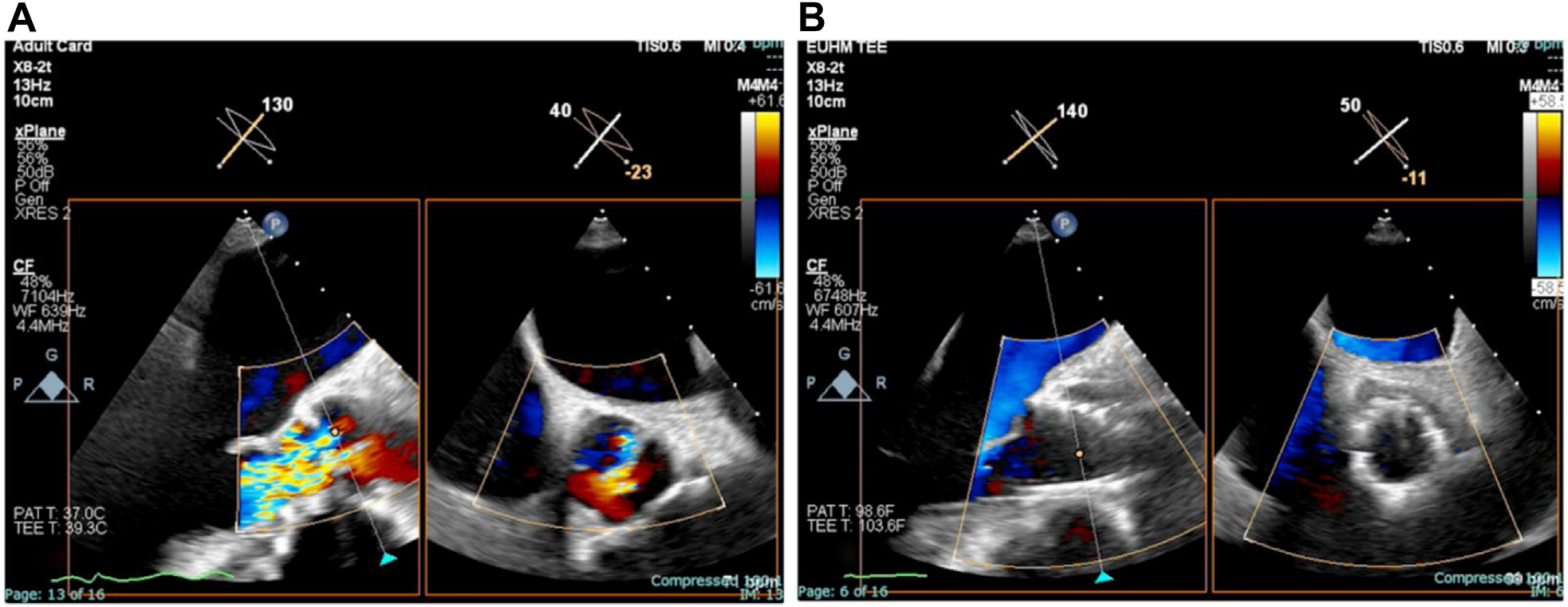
Transesophageal echocardiography images before and after intervention. (A) Severe aortic insufficiency visualized on short and long axes using transesophageal echocardiogram preprocedurally. (B) Postprocedural reduction of aortic valve insufficiency from severe to none.
